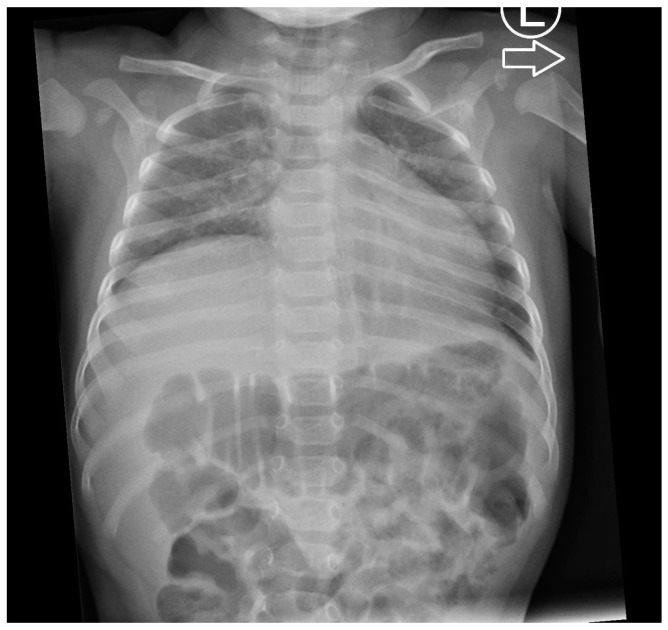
A chest X-ray of the patient no. 3 with right-sided eventration of the diaphragm at 4 months.
